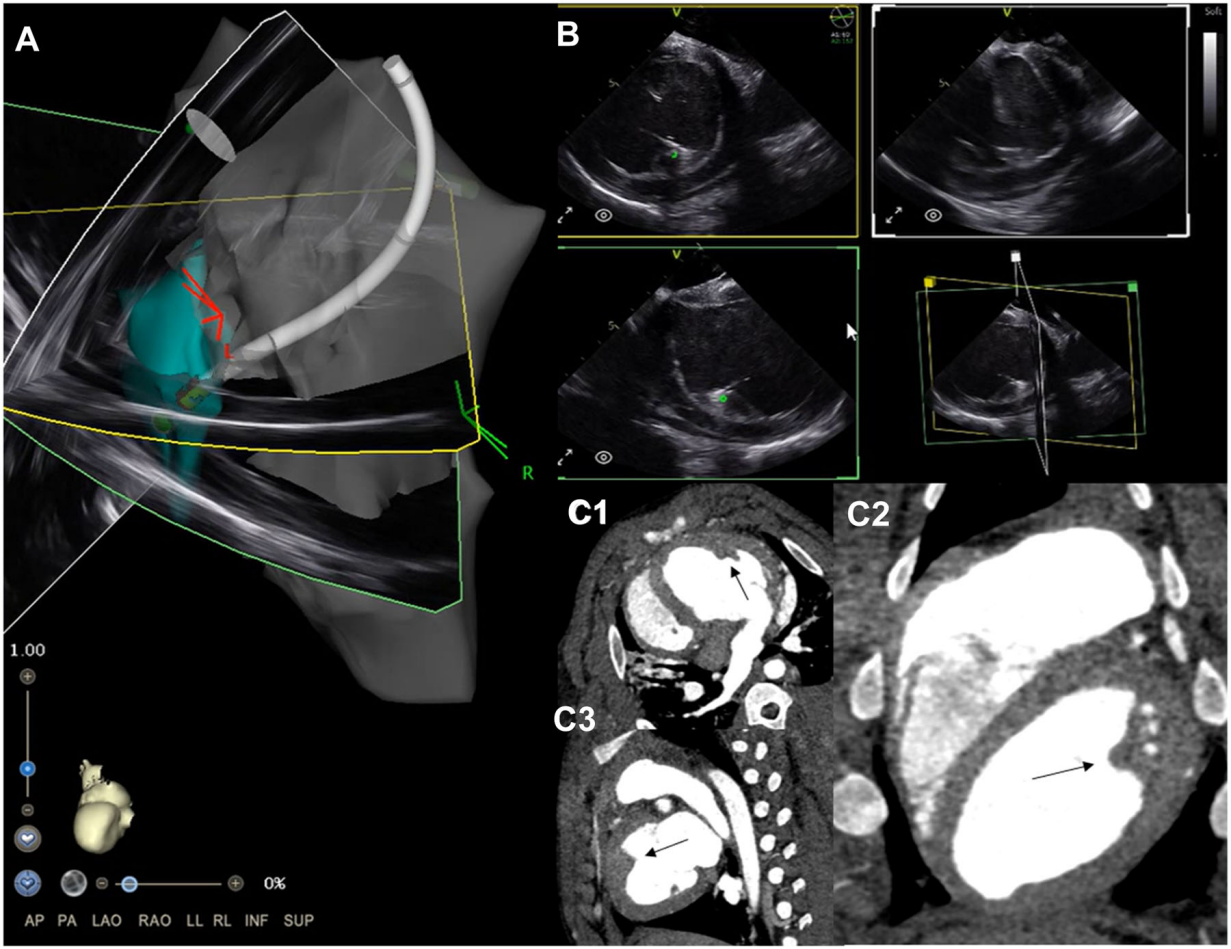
4D ICE guiding ablation of the anterior papillary muscle using Carto (A), multiplane images from the 4D ICE catheter showing localization of the ablation catheter tip (green dot) on the papillary muscle in multiple views (B), and CT images showing the structure of the papillary muscle on CT compared to 4D ICE in the transverse (C1), coronal (C2), and sagital (C3) planes.
